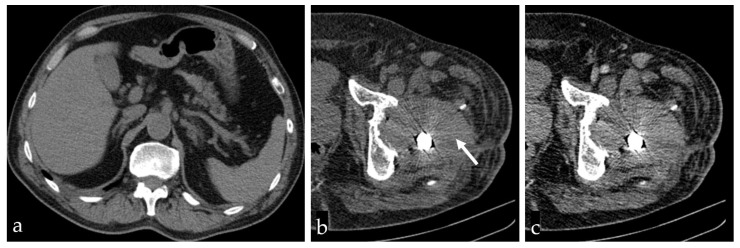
Computed Tomography scan showing normal size of spleen (a), recent periprosthetic hematoma with densities of approximately 60–80 HU (b, arrow), and no active bleeding, i.e., no pathological enhancement with contrast injection (c).
